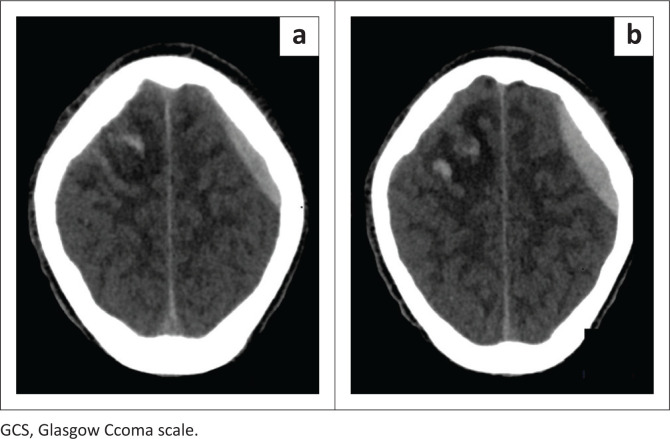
Seventeen-year-old male, post assault with a blunt object with an initial Glasgow Coma Scale score of 15 on presentation. Axial CT brain unenhanced on brain setting (a, b) demonstrates right frontal subarachnoid haemorrhage (a), interhemispheric subdural haemorrhage (a, b), right frontal intraparenchymal haemorrhage (b), perilesional oedema (a, b), left frontal and parietal extra dural haemorrhage (a, b).
